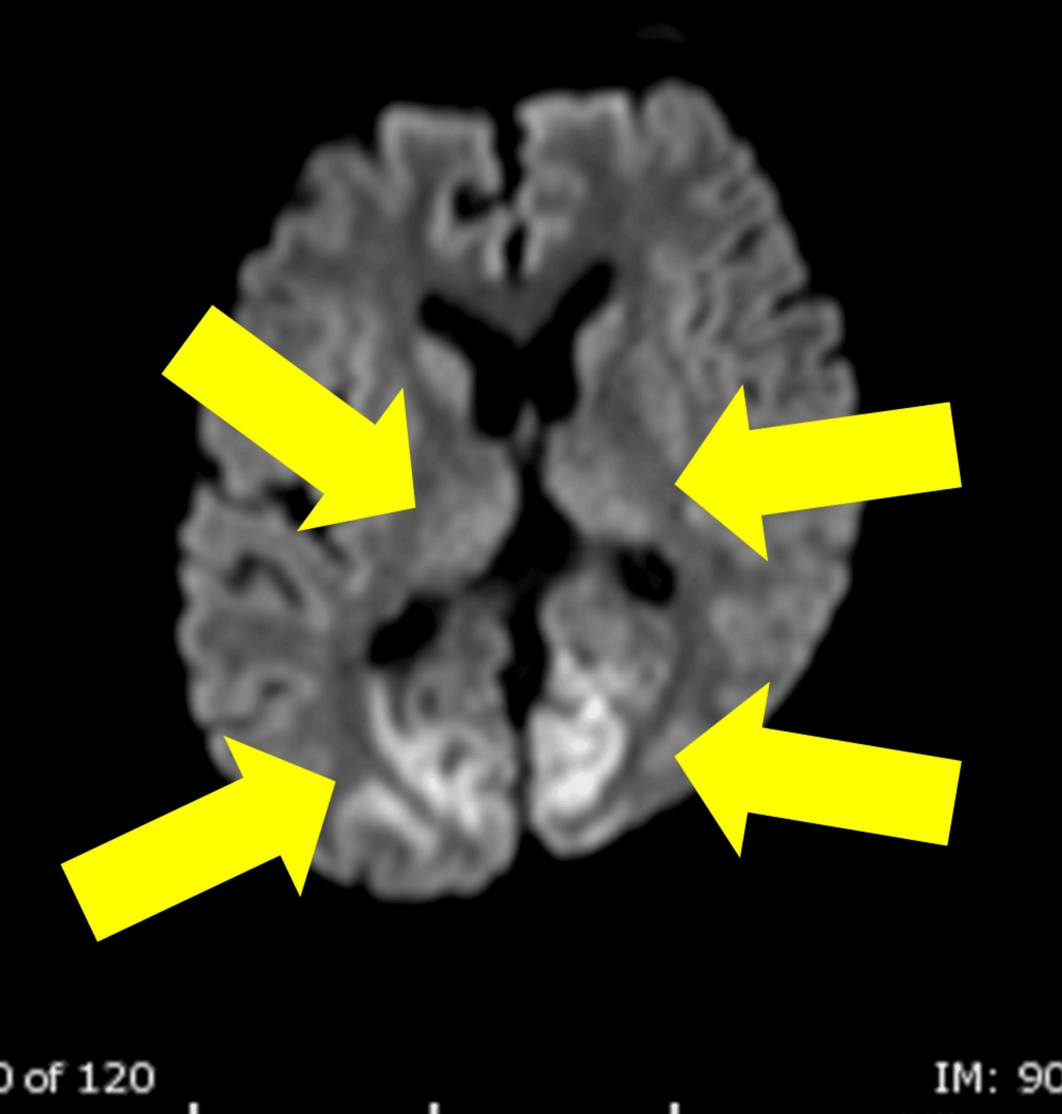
MRI image of the head; DWI high signals were observed in the bilateral occipital and medial thalamus DWI: diffusion-weighted imaging
